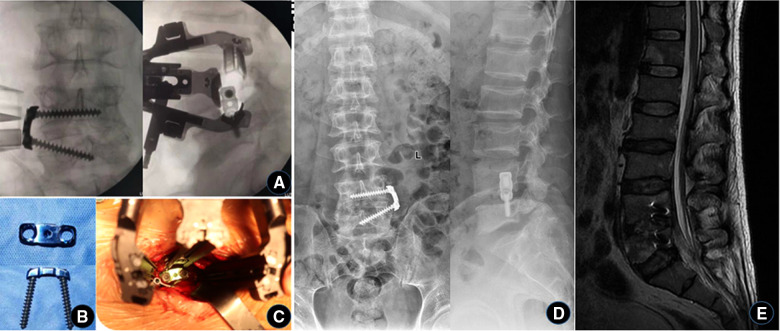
The third stage of iliac bone grafting and internal fixation. (A–C) During the operation, the iliac bone block was taken from the original incision, placed in the intervertebral space, and then fixed with a self-designed lateral lumbar spine plate. (D) X-ray and (E) MRI were reviewed 1 week after operation, showing that the internal fixation was well in place and the abscess in the spinal canal disappeared.
